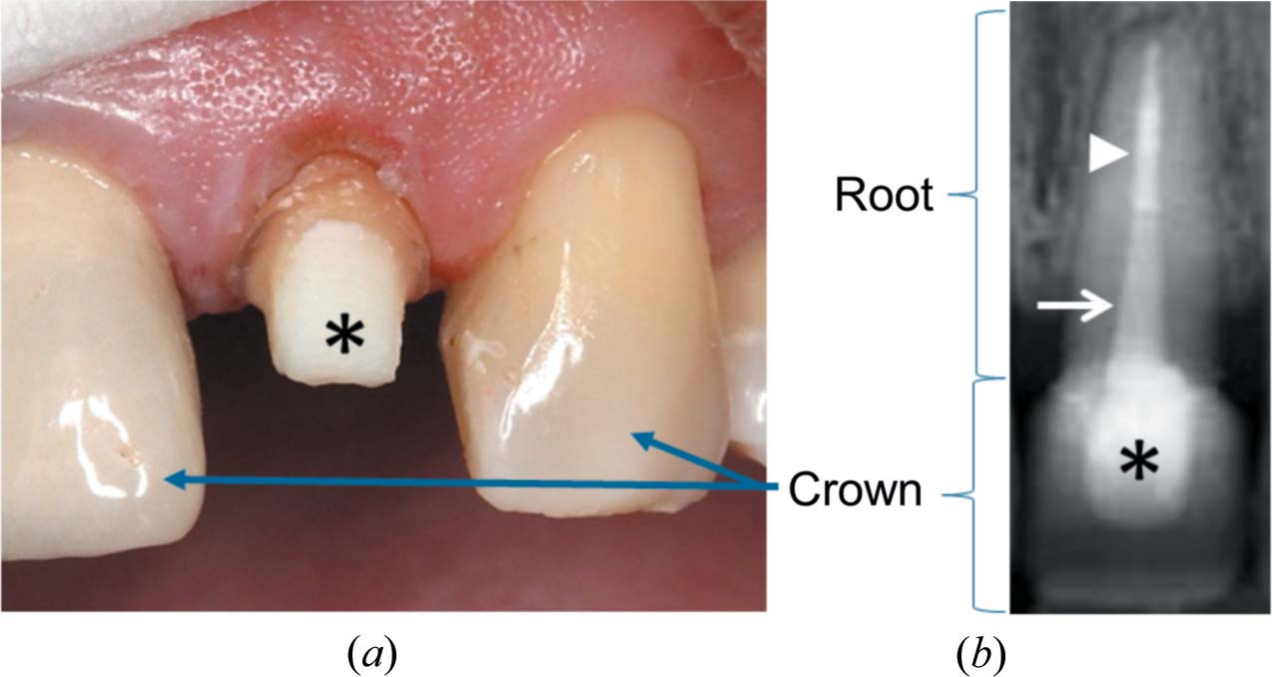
(*a*) Intra-oral photograph showing a tooth with a core (*), which is the only visible part of the post-and-core restoration during treatment, prior to coverage with a crown. (*b*) Radiograph of a similar tooth in which the core (*) is seen beneath an artificial crown, supported by a fibreglass post (white arrow) that is cemented into the disinfected and sealed (white arrowhead) root canal. (Clinical photographs courtesy of Drs Nestor Tzimpoulas and Wesley Thé, The Netherlands.)
